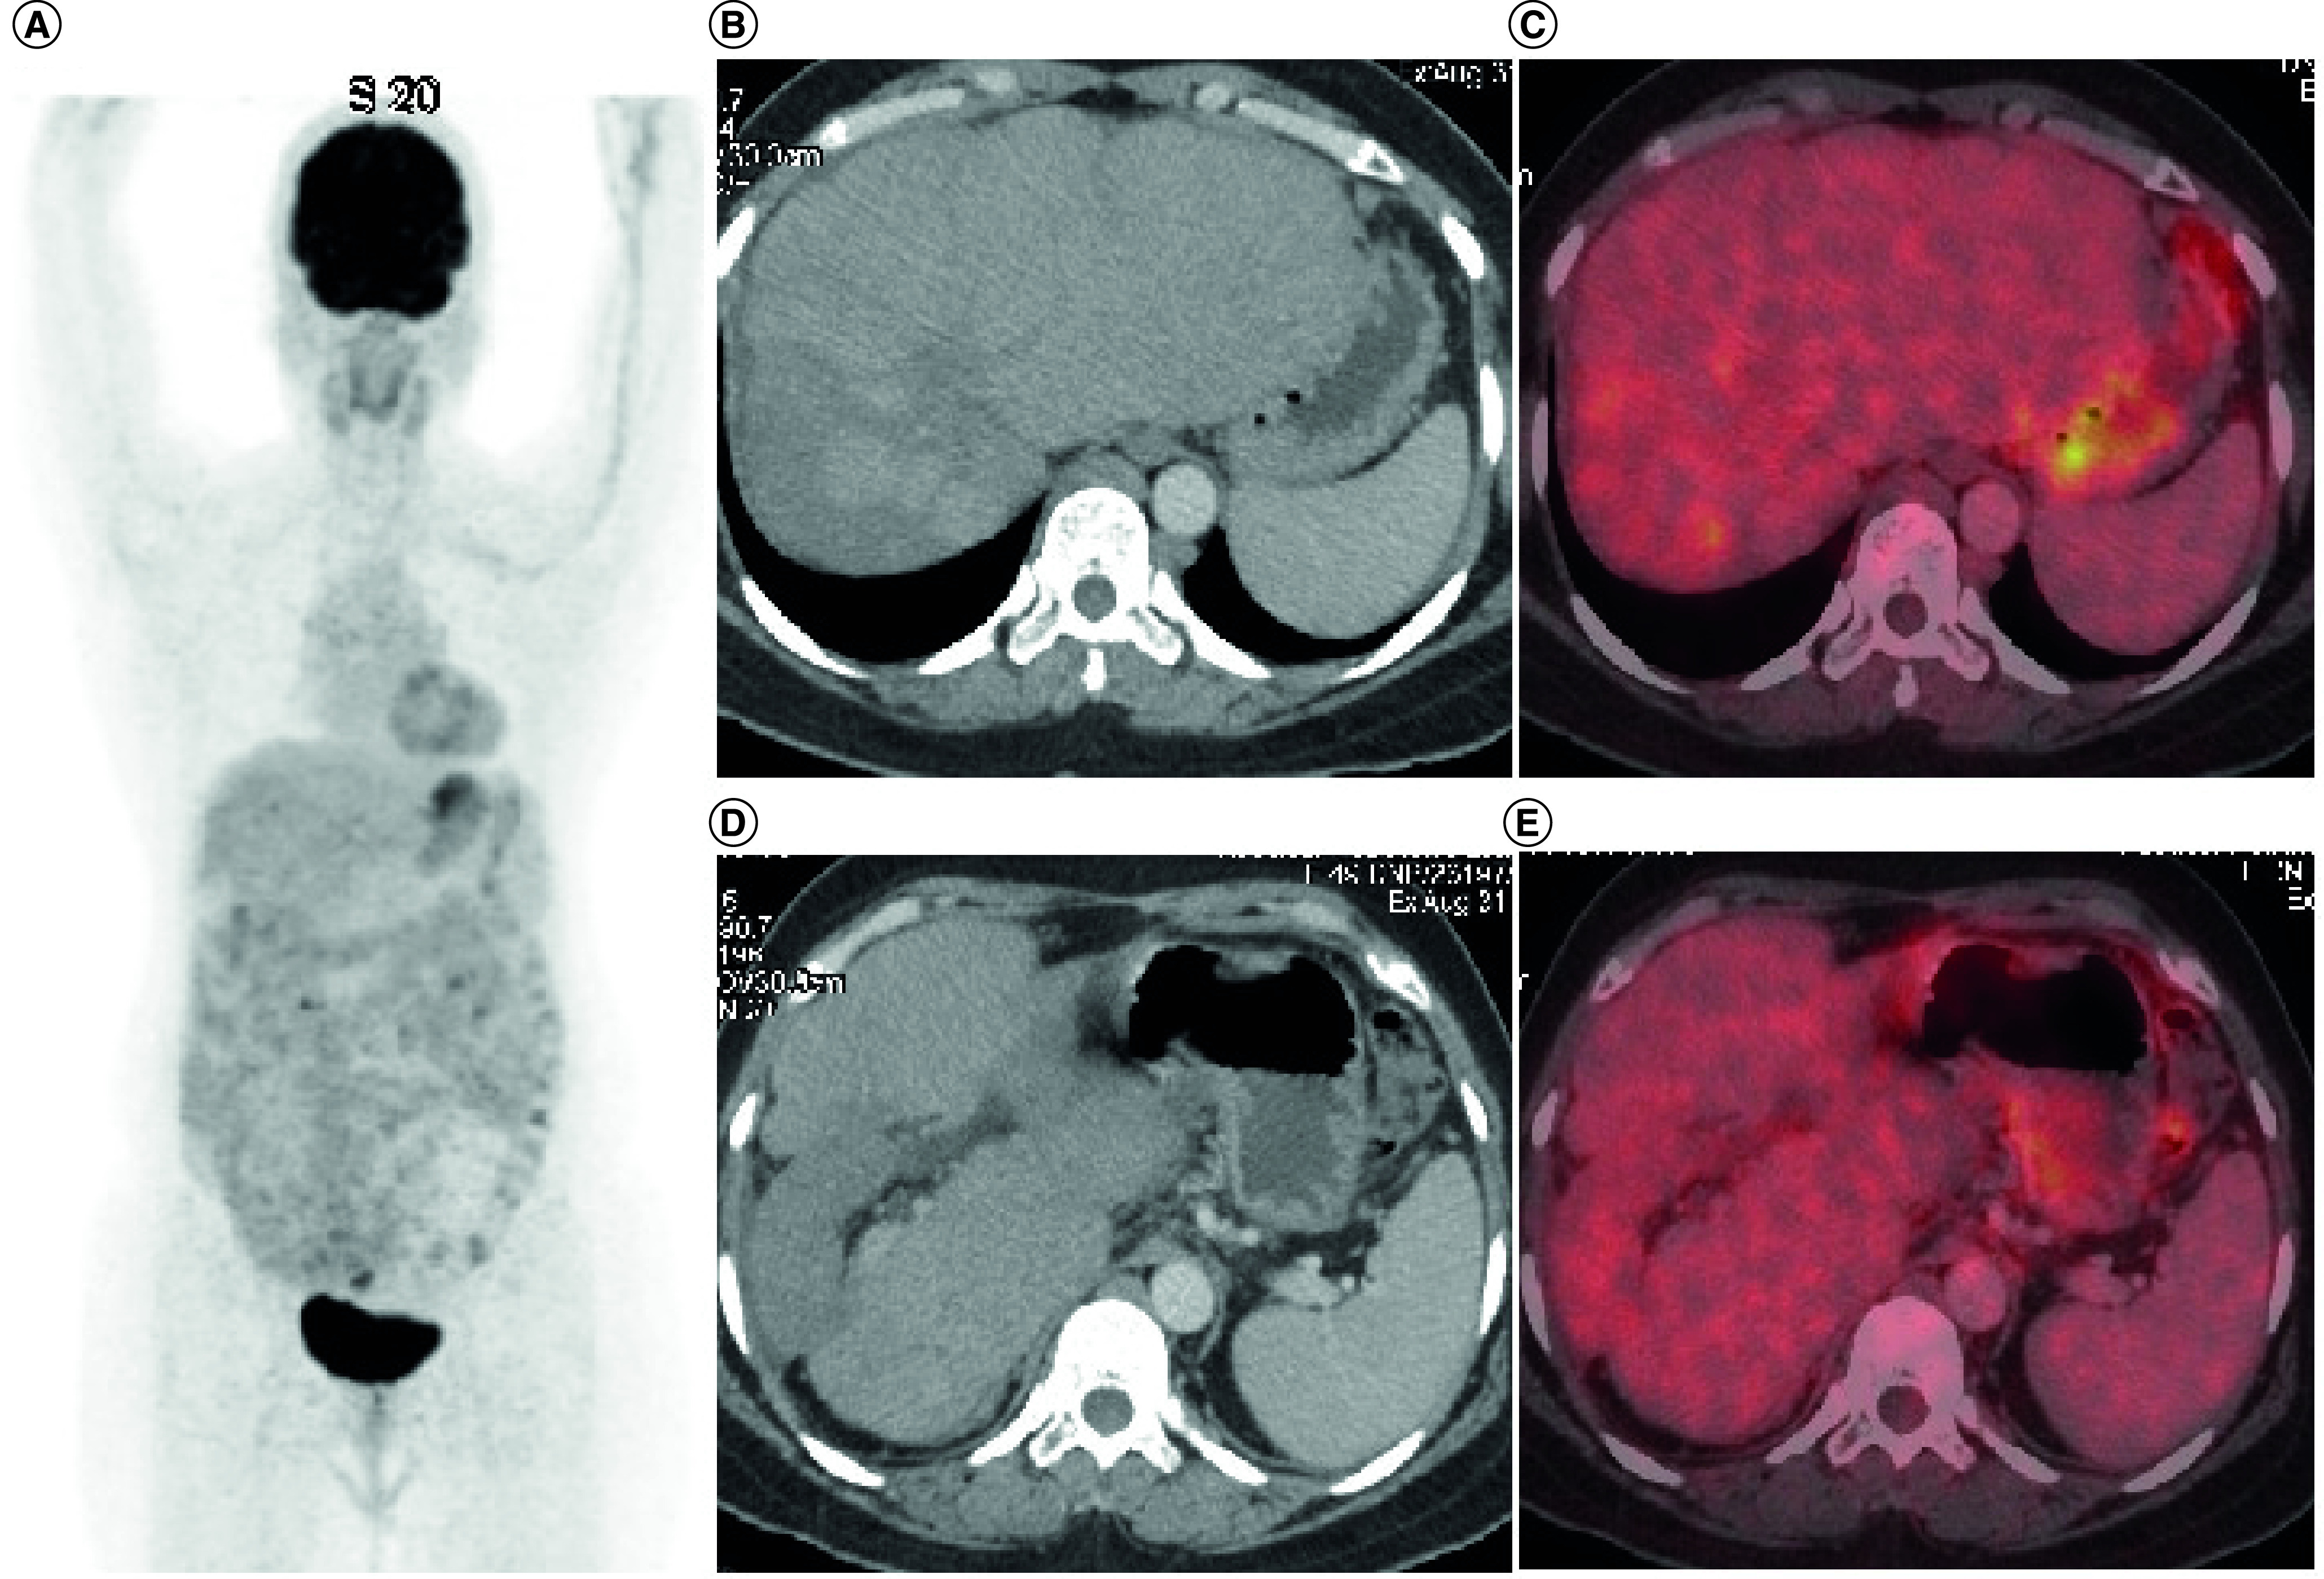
Post-treatment positron emission tomography-computed tomography scan of the 49-year-old female patient described in Figure 2 after 12 months of pazopanib-based therapy. (A) Maximum intensity projection image FDG PET-CT showing physiologic tracer uptake in brain, myocardium and urinary bladder and no abnormal tracer uptake in the hepatic region. (B & D) Axial CT abdomen showing confluent hypodense lesions in both the lobes of liver. (C & E) There is no significant abnormal tracer uptake in fused PET-CT images suggestive of complete metabolic response.
